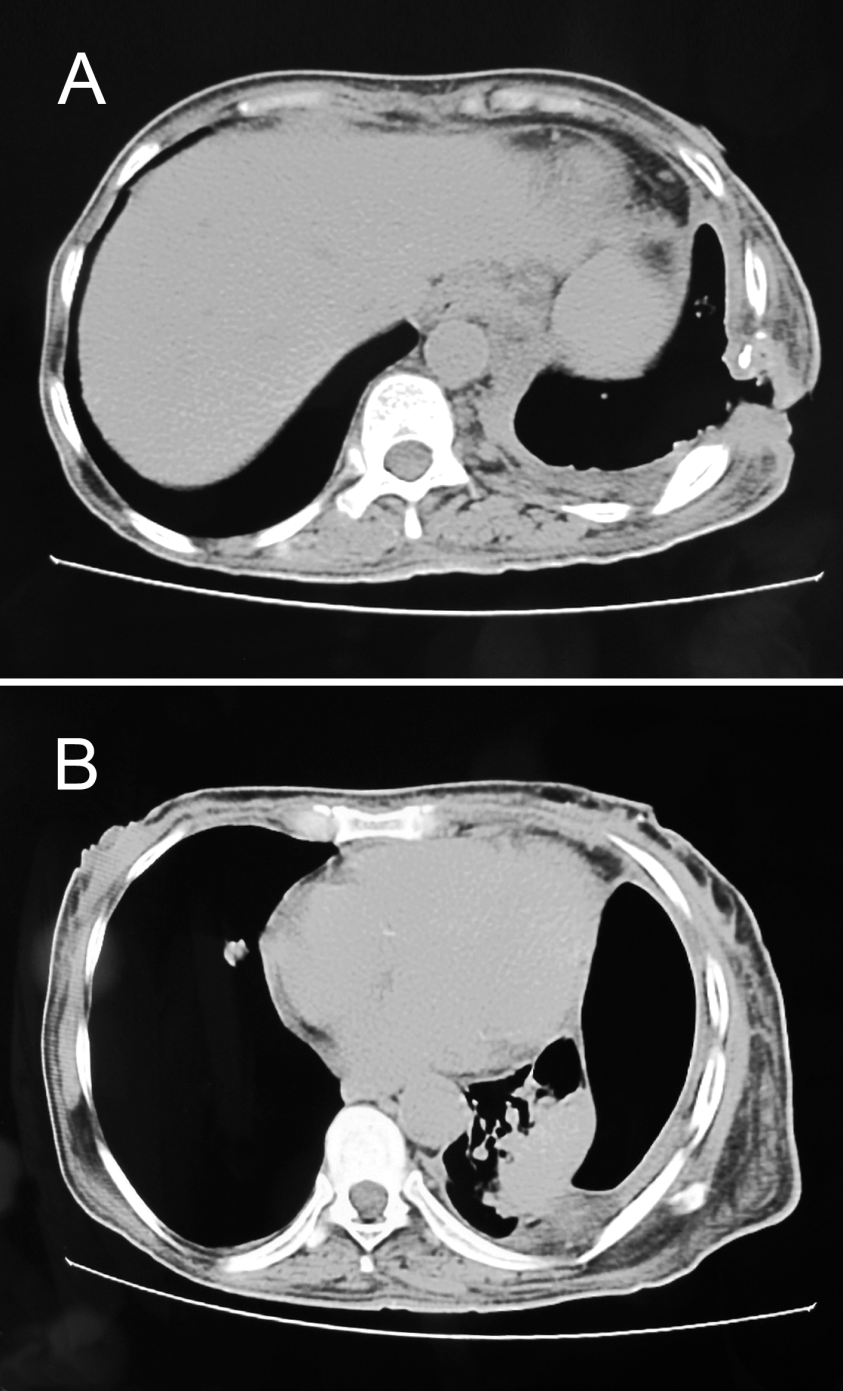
Chest computed tomography (CT) scan showing (A) left lower chest wall thickening with a wall defect and (B) left side hydropneumothorax with lower lobe collapse and consolidation of the lingual.
